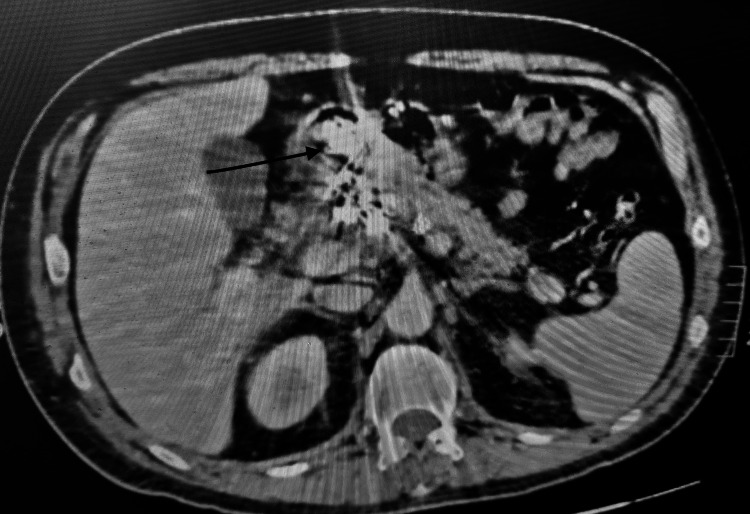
Contrast-enhanced CT axial view depicted the pyloric erosion and protrusion of coils in the proximal part of the duodenum (black arrow). CT: computed tomography
